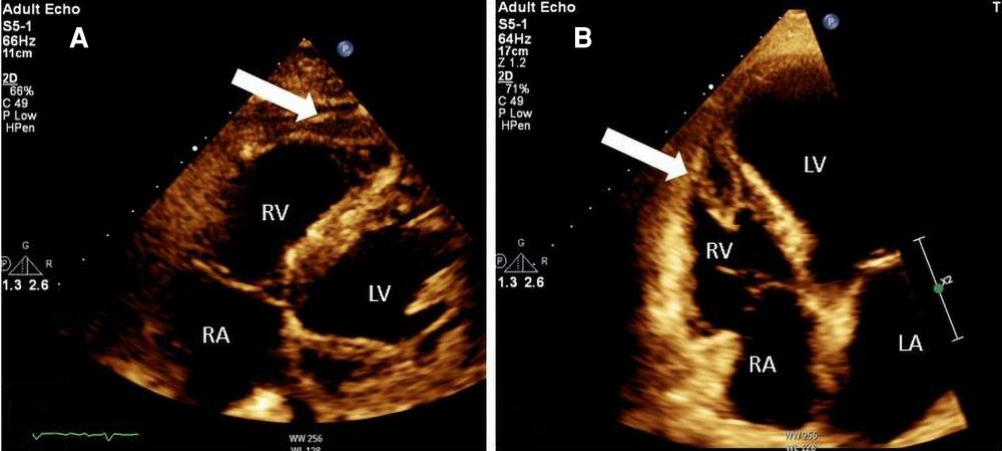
Transthoracic Echocardiography, right ventricular focused view (*A*) and an apical four-chamber (*B*) showing a significant reduction in the mass size (white arrows).
